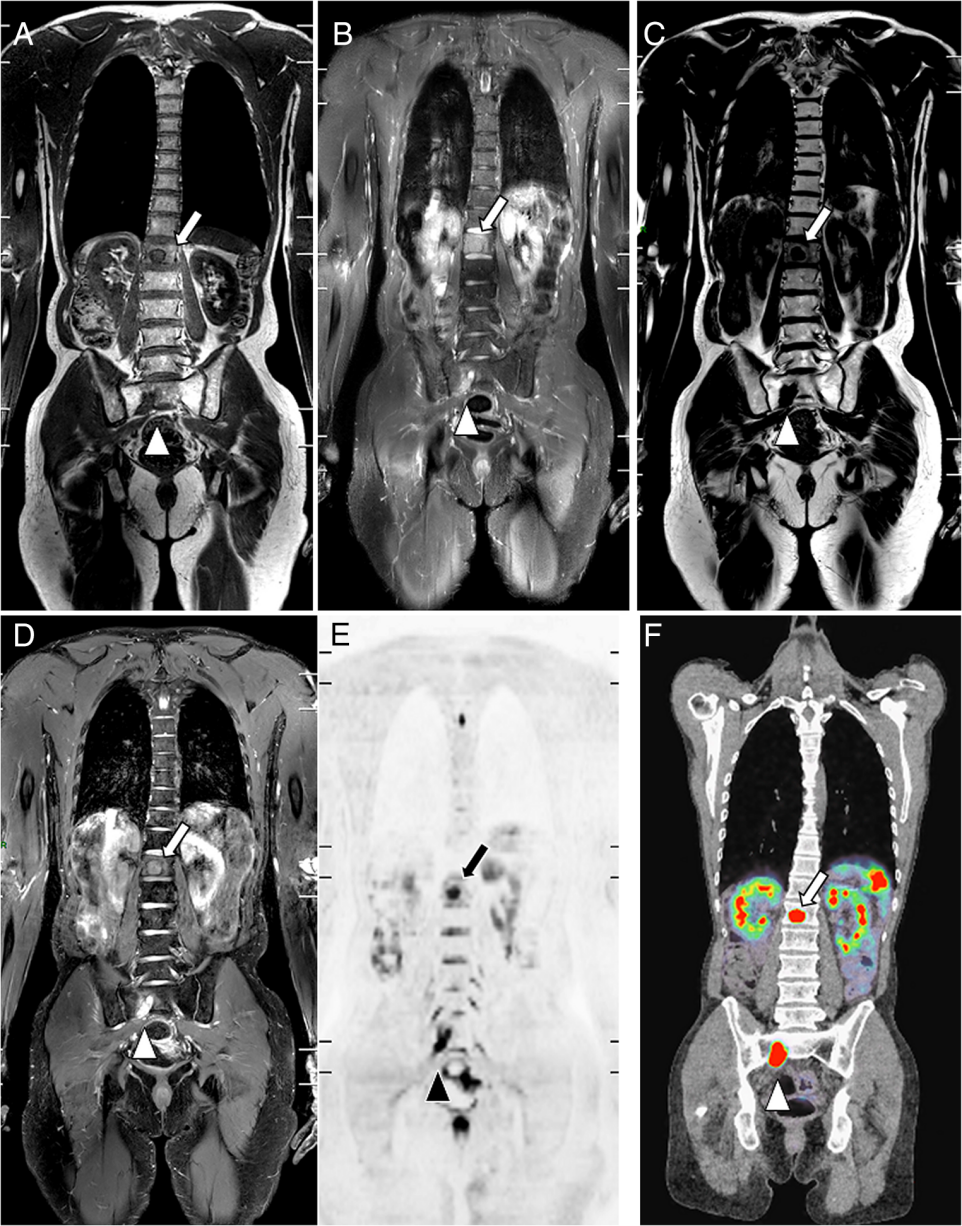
Agreement between sequences on metastatic lesions: WB-MRI study in a 46-year-old man with metastatic neuroendocrine cancer. Two metastases are observed in the L1 vertebral body (arrow) and in the right wing of the sacrum (arrowhead). Coronal T1 (A) and STIR (B) WB-MRI images show both lesions. Fat (C) and water (D) reconstructions of the TSE T2 Dixon acquisition show the same lesions. The reference standard, based on reading of all MR images and concurrent imaging studies, confirmed the presence of two metastases. E Concurrent DWI image (*b* = 1000 s mm^−2^, inverted gray scale) confirms the presence of both lesions and shows no additional lesion. F Gallium-68 dotatate PET/CT fused image shows the same two lesions presenting tracer uptake
